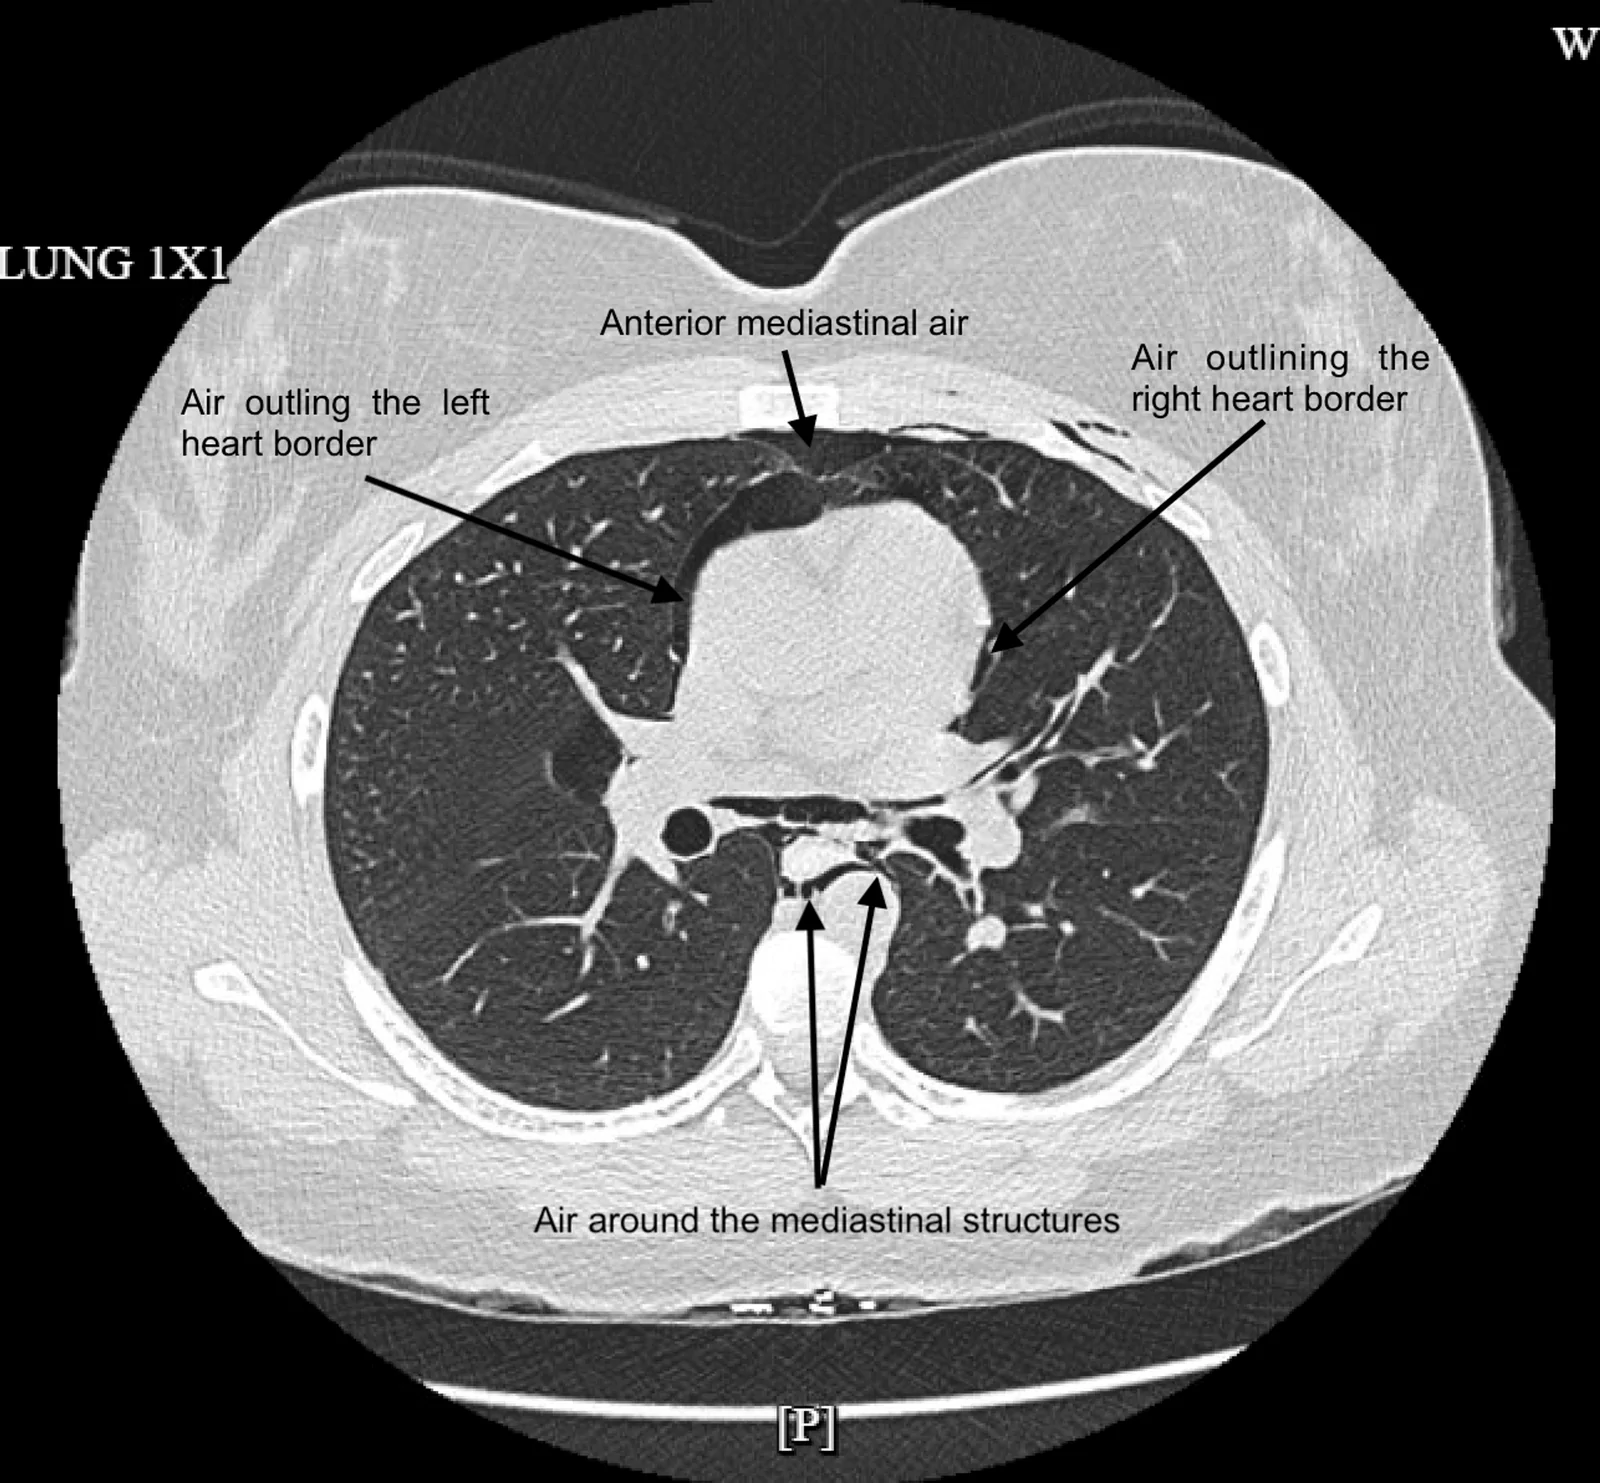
CT chest without contrast showing extensive pneumomediastinum with small pneumopericardium anteriorly and extensive subcutaneous emphysema in the anterior thorax.
